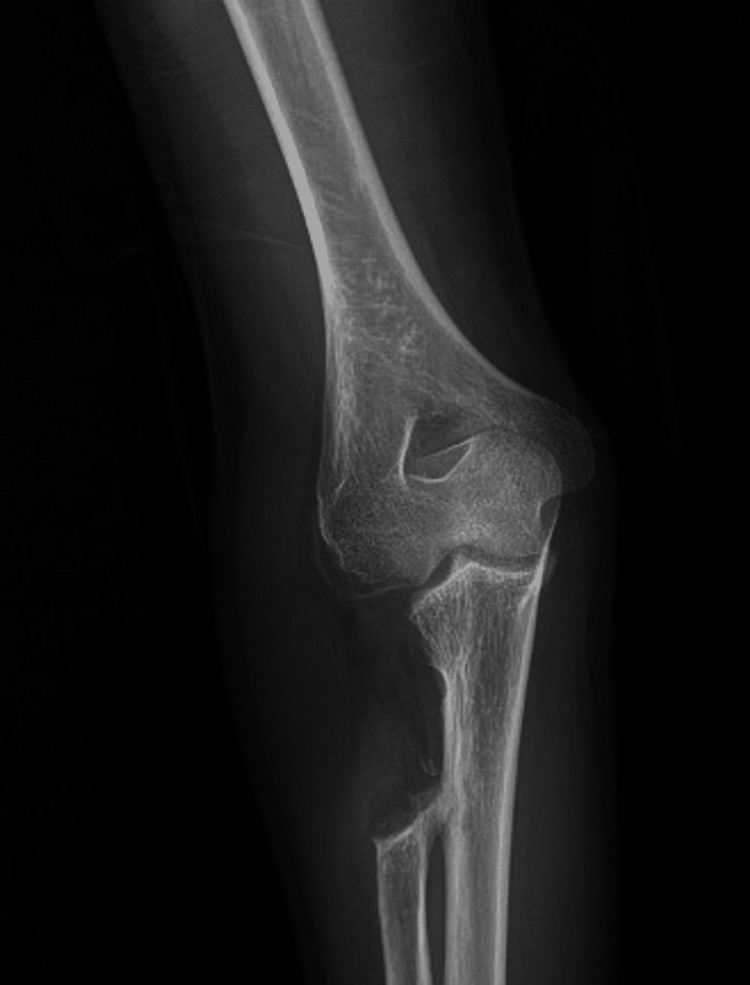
One-year postoperative plain radiograph. One-year postoperative plain radiograph of the right elbow joint showed mild elbow valgus deformity with no radial shaft proximal migration or tumor recurrence.
